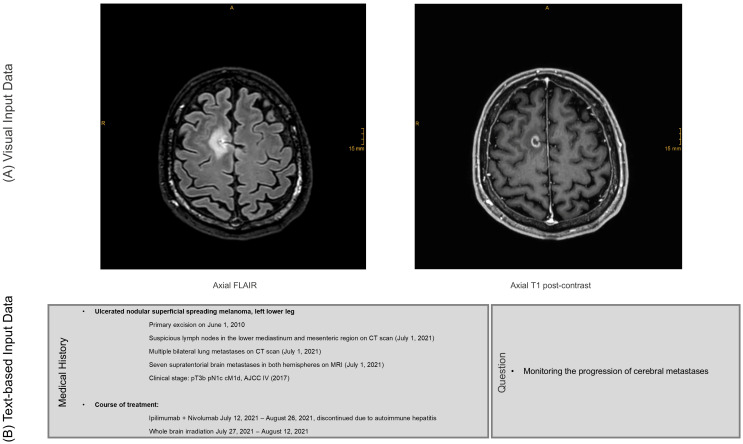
Input Data for AI-Generated Radiology Reports. (A) Visual input: MRI images consisting of single-slice axial FLAIR and contrast-enhanced T1-weighted sequences, including a visible scale reference to allow for size estimation. (B) Textual input: Clinical background information, including the patient’s medical history, relevant clinical details and the referring physician’s question. Both the imaging data and clinical information were provided to the AI models to enable report generation under conditions resembling routine clinical practice. Abbreviations: FLAIR—fluid-attenuated inversion recovery; AI—artificial intelligence.
